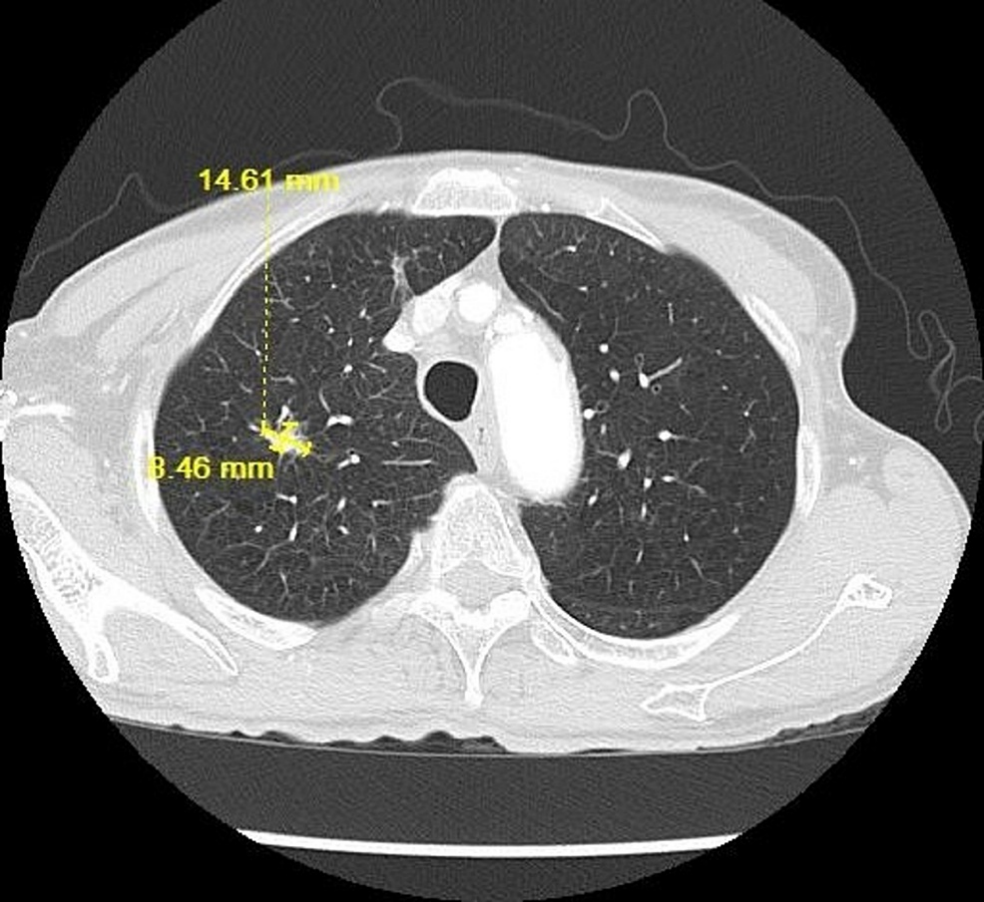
CT chest lung window showing the increased size of the lung nodule to 14.61 x 8.46 mm on 03/12/2018
